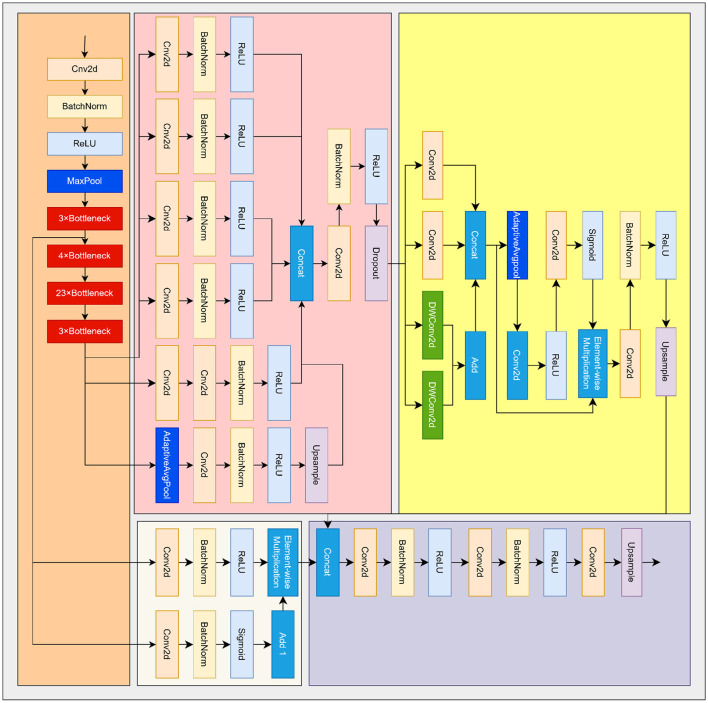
Overall architecture of the proposed DPF–EHDNet. The network adopts a DeepLab-style encoder-decoder backbone and integrates three thyroid-oriented modules: (1) the Dual-Path Differential Feature (DPF) module enhances discriminative structural responses by learnable high- and low-frequency decomposition; (2) the Edge-aware Atrous Spatial Pyramid Pooling with Enhancement (ASPP-E) module aggregates multi-scale semantic context while preserving structurally relevant cues; and (3) the Edge-Guided Hybrid Decoder (EHD) selectively fuses reliable shallow features using an edge-derived confidence map. Together, these components improve robustness to speckle noise, heterogeneous echogenicity, and structural ambiguity commonly encountered in thyroid ultrasound imaging.
